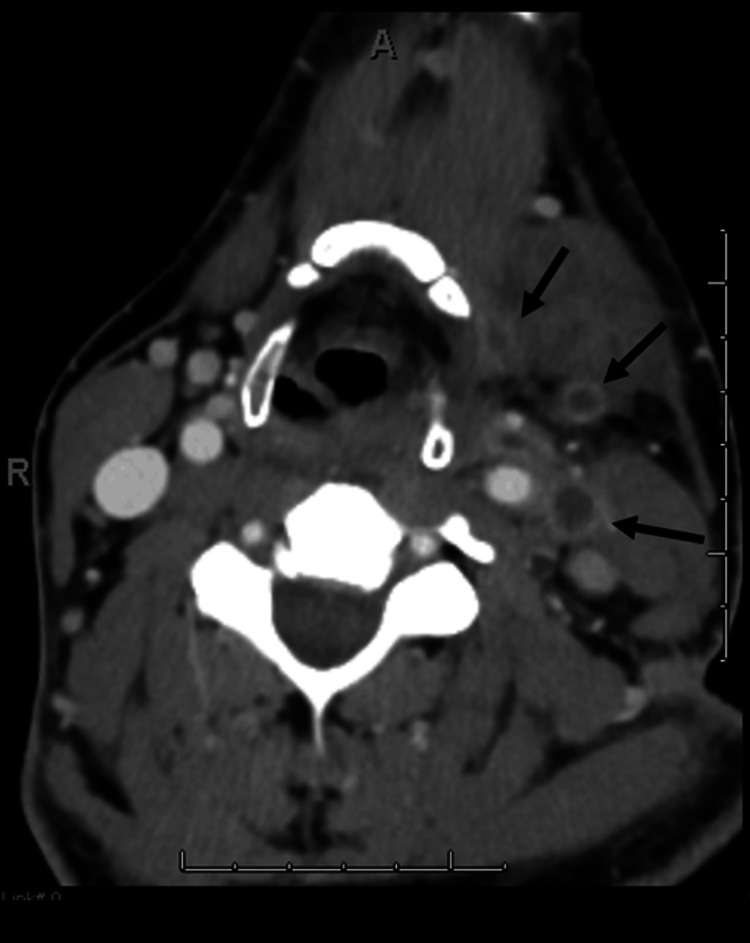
Repeat axial contrast-enhanced CT showing complete occlusion of the facial, lingual, and anterior jugular veins, indicated by the black arrow.
